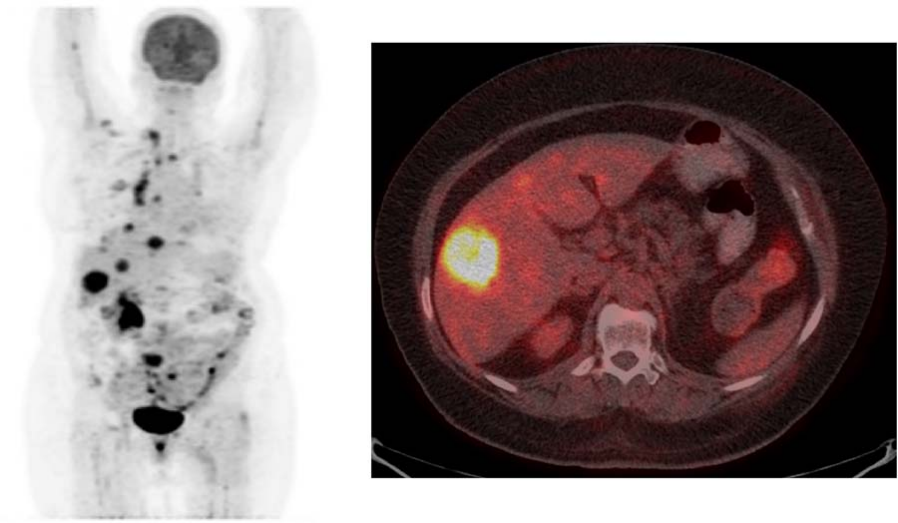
Whole-body maximum-intensity-projection (MIP) PET showed abnormal FDG uptake in the right infraclavicular area, mediastinal lymph nodes, liver and skeletal system suggesting widespread metastasis in the patient. Axial section of the liver on fused PET-CT showed abnormal intense FDG uptake (SUVmax: 15.8).
